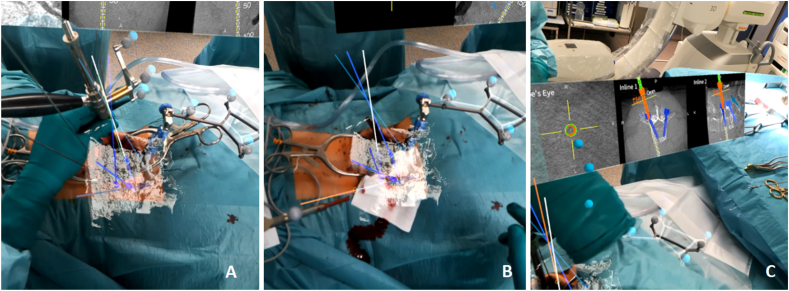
Exemplary case of lumbar spine surgery with surgeon's view through the MagicLeap2 device: A) and B) mixed reality with spine model and screw planning and their trajectories in L3, L4 and L5; C) augmented reality with visualization of CT scan with preplanned screws and real time navigated screw placement.
